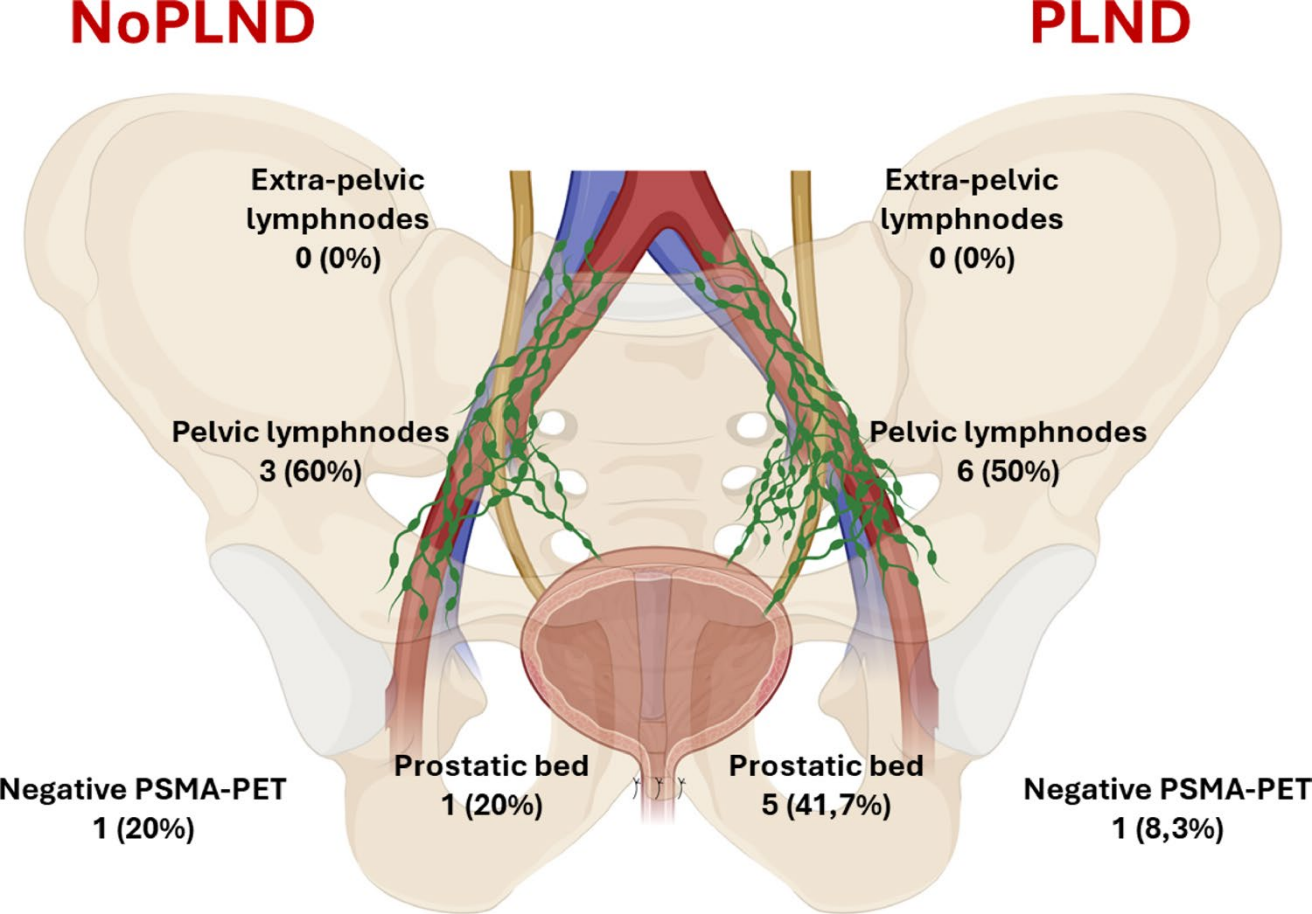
Distribution of recurrences detected with PSMA-PET/CT among patients with biochemical failure treated with pelvic lymph nodes dissection and patients treated with radical prostatectomy alone
